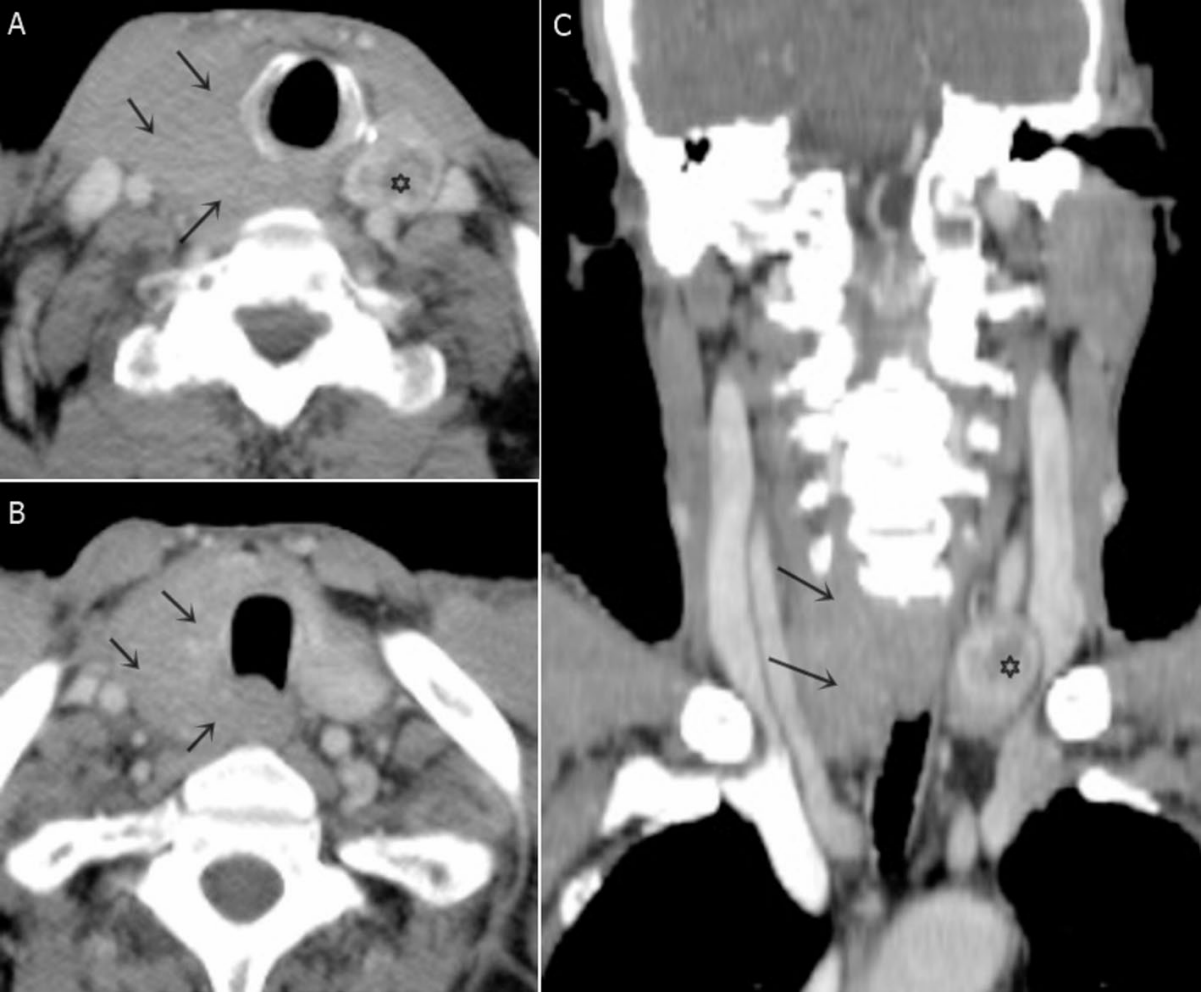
CT scan of a case of PTL: A 66-year-old male with non-Hodgkin lymphoma (DLBC type) in the right thyroid lobe. Post-contrast CT neck axial (A & B) and reconstructed coronal (C) images revealed infiltrative hypo-enhancing right thyroid lobe mass (arrows) measures 5.5 × 3 × 9 cm, it’s seen inseparable from Rt sternomastoid muscle laterally, contacting both right common carotid artery (CCA), right internal jugular vein (IJV) without caliber changes and extending into post cricoid region posteromedially. No detected tracheal deviation. A small left thyroid hypodense nodule (asterisk) measures 1 cm. No detected suspicious cervical LNs
